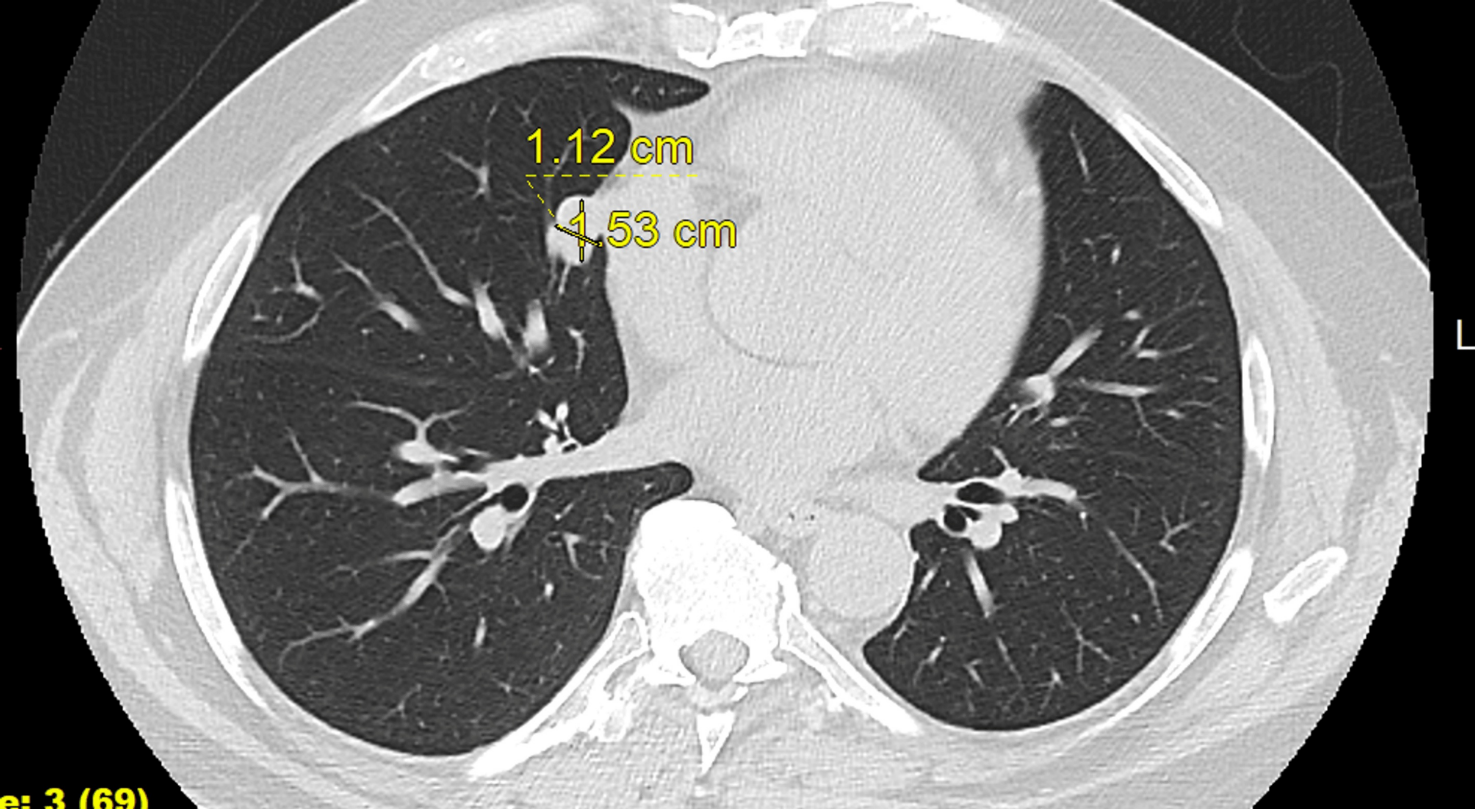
Chest CT of a 1.5 cm right middle lobe pulmonary nodule
